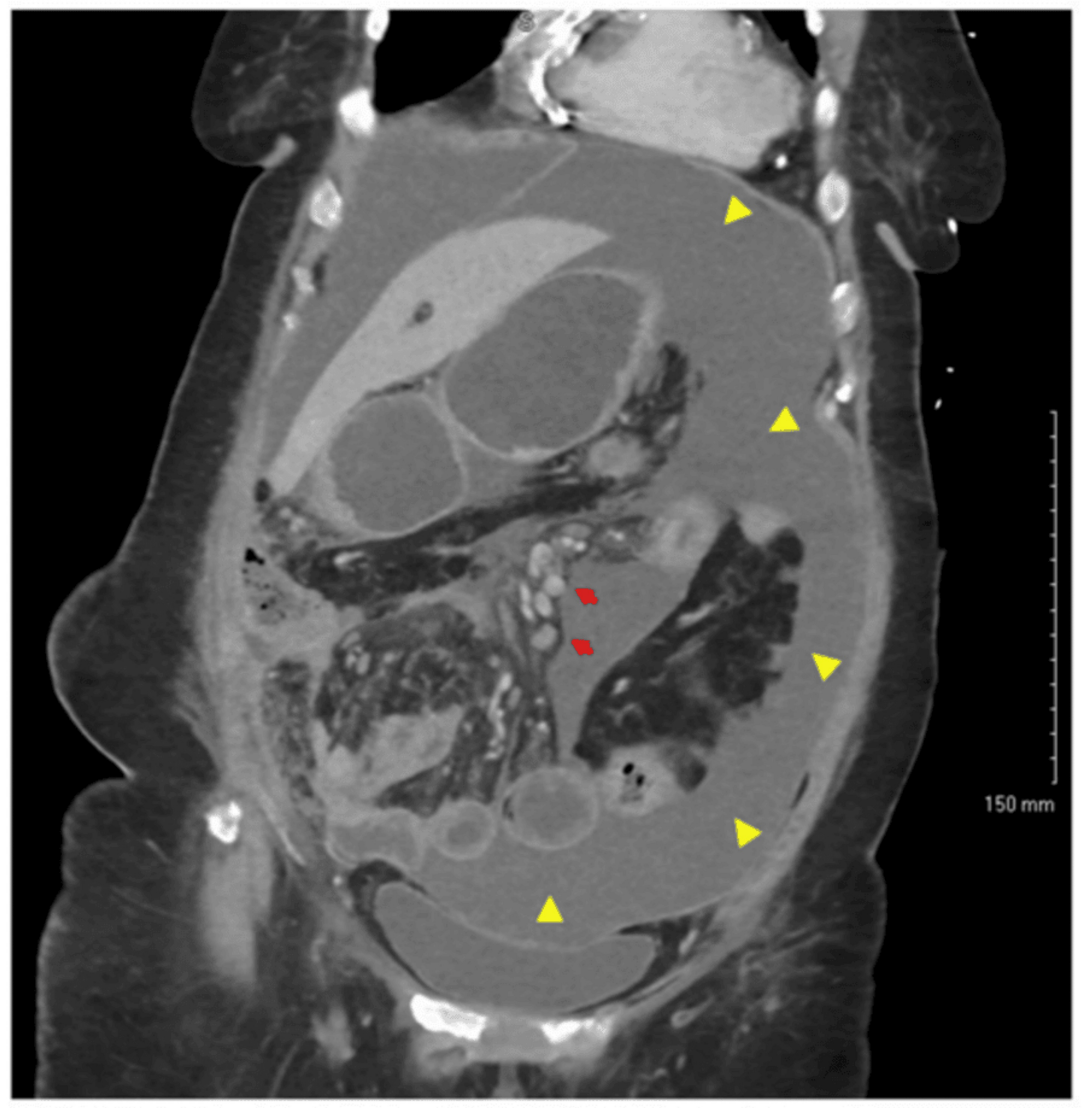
CT of the abdomen and pelvis with contrast Known peritoneal carcinomatosis. Moderate amount of ascites (yellow arrowheads) and retroperitoneal and mesenteric lymphadenopathy (red arrows). Mildly dilated loops of small bowel suspected partial small bowel obstruction. CT: computed tomography
